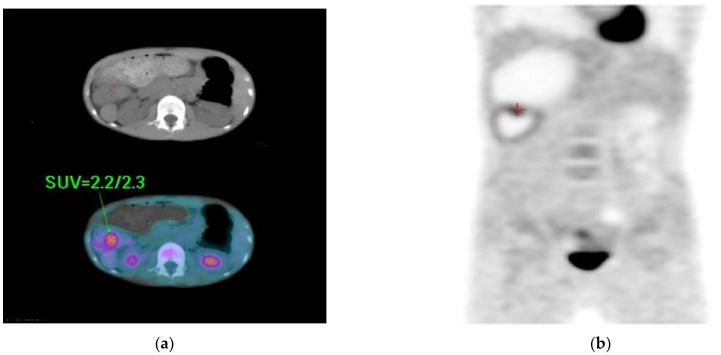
Whole-body PET scan showing: (a,b) persistent focal increase in FDG uptake in the right upper abdomen (early/delayed SUV max = 2.2/2.3) and a tumor or inflammation was pointed out <red duplex and arrow>. SUV = standard uptake volume.
